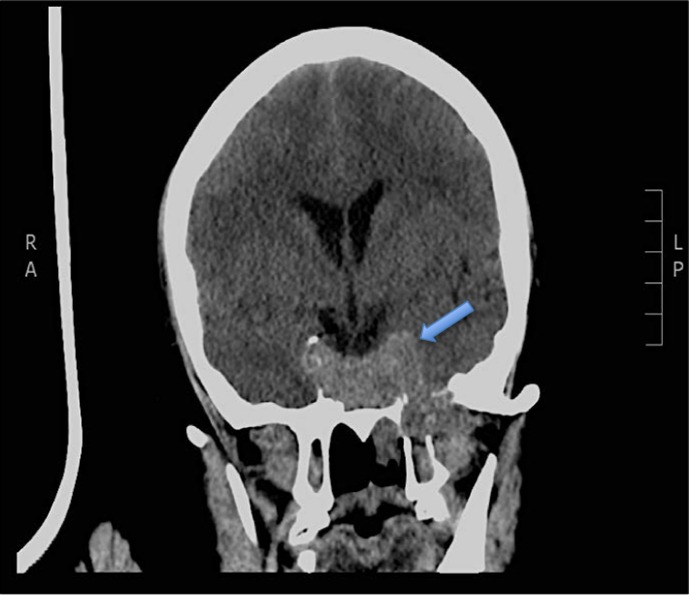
Computed tomography brain scan that revealed a parenchymal mass at the base of the skull eroding the body of the clivus (blue arrow).
